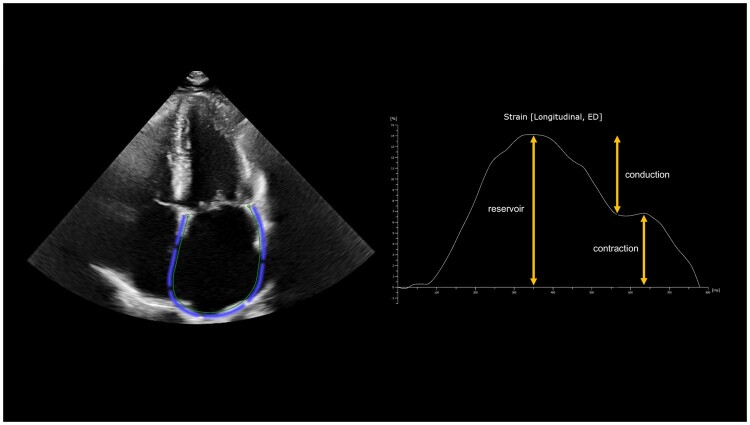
Example of left atrial tracing in the apical 4-chamber view followed by left atrial strain analysis.
